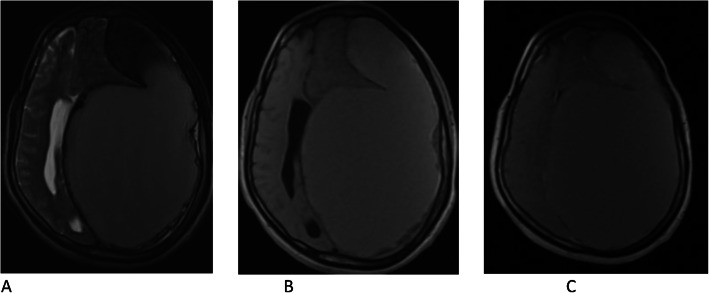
Pre- and postcontrast magnetic resonance imaging. a Axial T2-weighted image showing left frontoparietal convexity mixed intensity subdural collection with mass effect on the lateral ventricles. b Axial T1-weighted image showing slightly hyperintense signal involving the same location. c Axial T1-weighted postcontrast images showing no abnormal contrast enhancement
